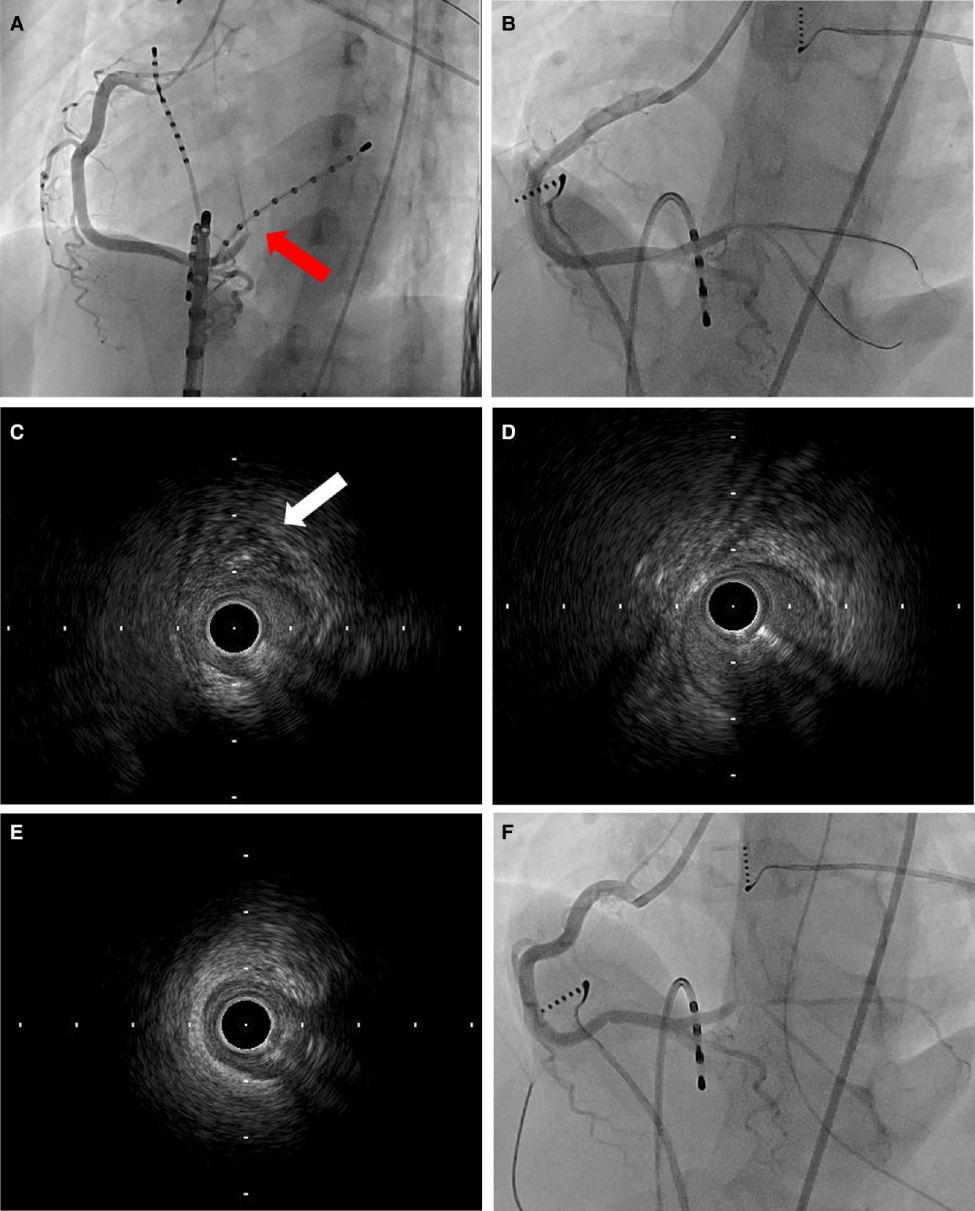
Coronary angiography and intravascular ultrasound imaging. (*A*) Occlusion of the dominant right coronary artery at the segment 4 atrioventricular branch (arrow). (*B*) Revascularisation following the passage of a floppy wire. (*C*) The vessel is narrowed at the target lesion. An abnormal area of low echogenicity is detected in the adjacent myocardium (arrow). (*D*) The proximal site shows no evidence of plaque rupture and maintains a normal three-layered structure. (*E*) Distal site maintained a normal structure. (*F*) Final angiography.
